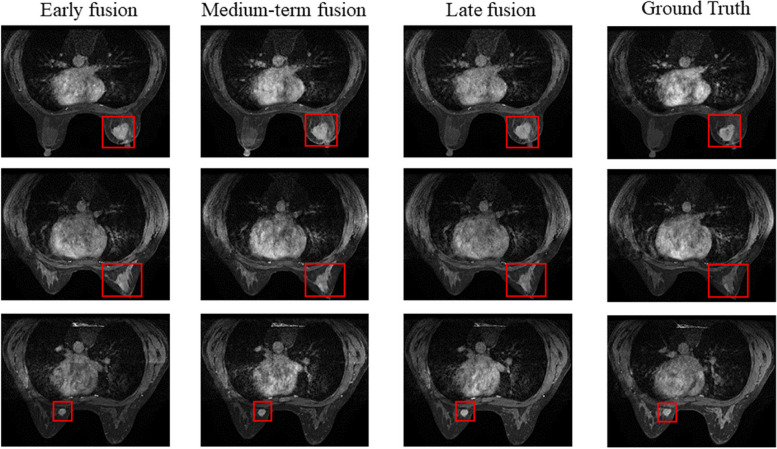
The generated eighth images of DCE-MRI using different way of feature fusion
